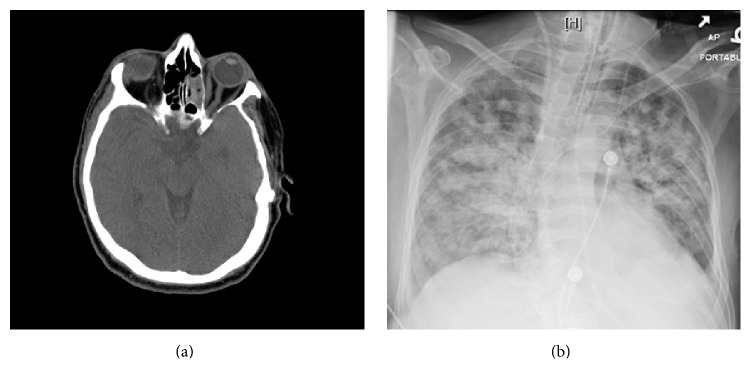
Radiologic findings. (a) Head CT scan shows fluid opacification consistent with inflammatory changes predominately in the left ethmoid sinus. No masses or bone destruction is seen. (b) Chest X-ray shows diffuse bilateral mixed interstitial and alveolar infiltrates.
